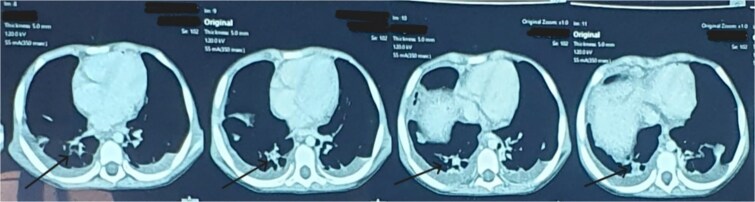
CT scan (axial view) showing moderate fluid collection in bilateral pleural cavities suggestive of bilateral pleural effusion (As shown by blue arrowheads).
